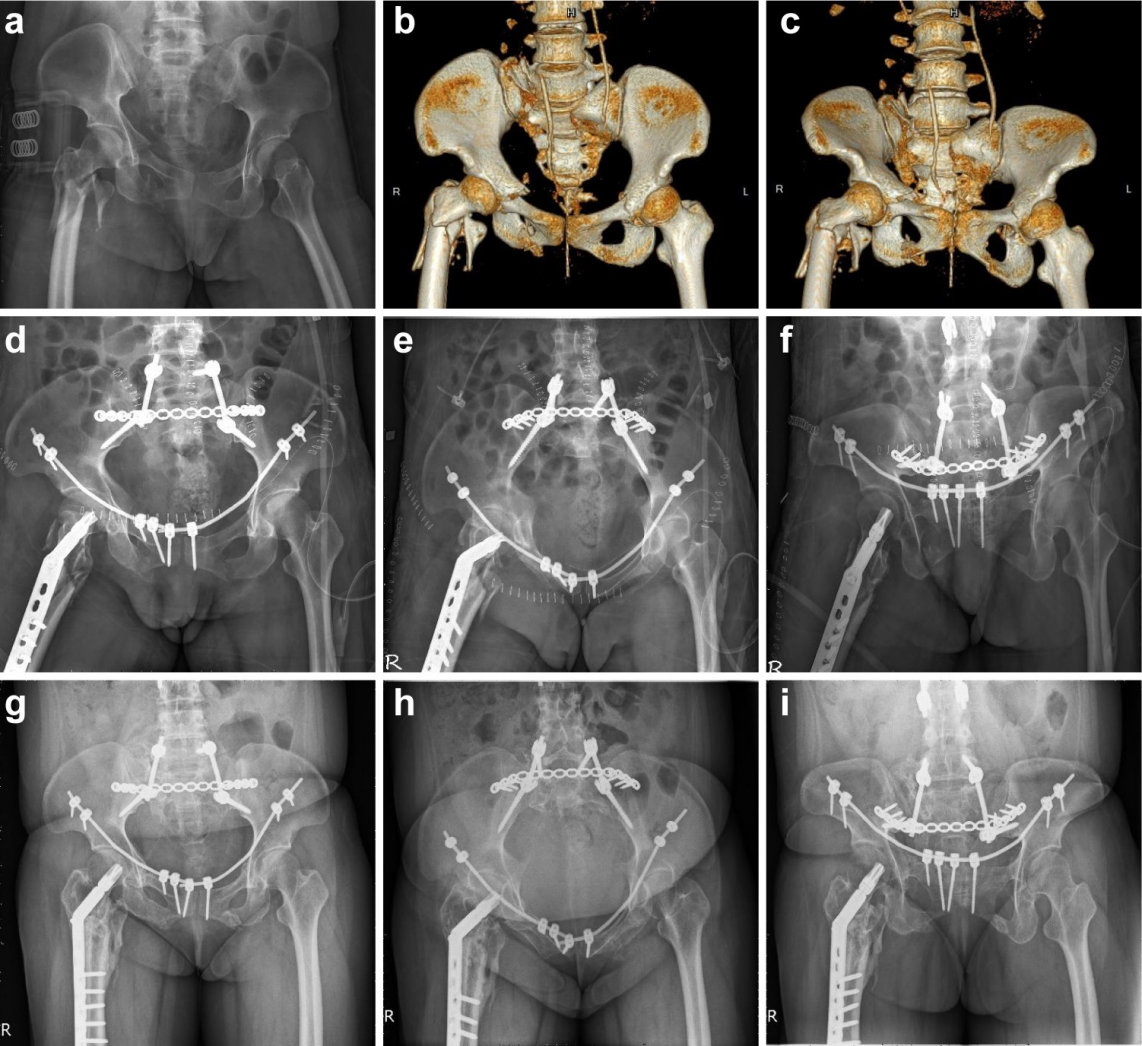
A 37-year-old female with anterior and posterior pelvic ring injury. a Preoperative pelvic anterior-posterior (AP) view. b Preoperative 3D reconstructed computed tomography (CT) AP view. c Preoperative 3D reconstructed CT outlet view showing a U-shape sacral fracture with bilateral superior and inferior pubic rami fractures and comminuted right proximal femur fracture. d, e, f Postoperative pelvic radiology series (AP, inlet, outlet view) demonstrating spinopelvic and transiliac plate fixation of the posterior pelvic ring fracture, bilateral MPSRF of the anterior pelvic ring fracture, and dynamic hip screw fixation of the right proximal femur. g, h, i Postoperative pelvic radiology series (AP, inlet, outlet view) at 13-month follow-up, demonstrating bone union of the pelvic ring. The quality of fracture reduction was graded as excellent.
